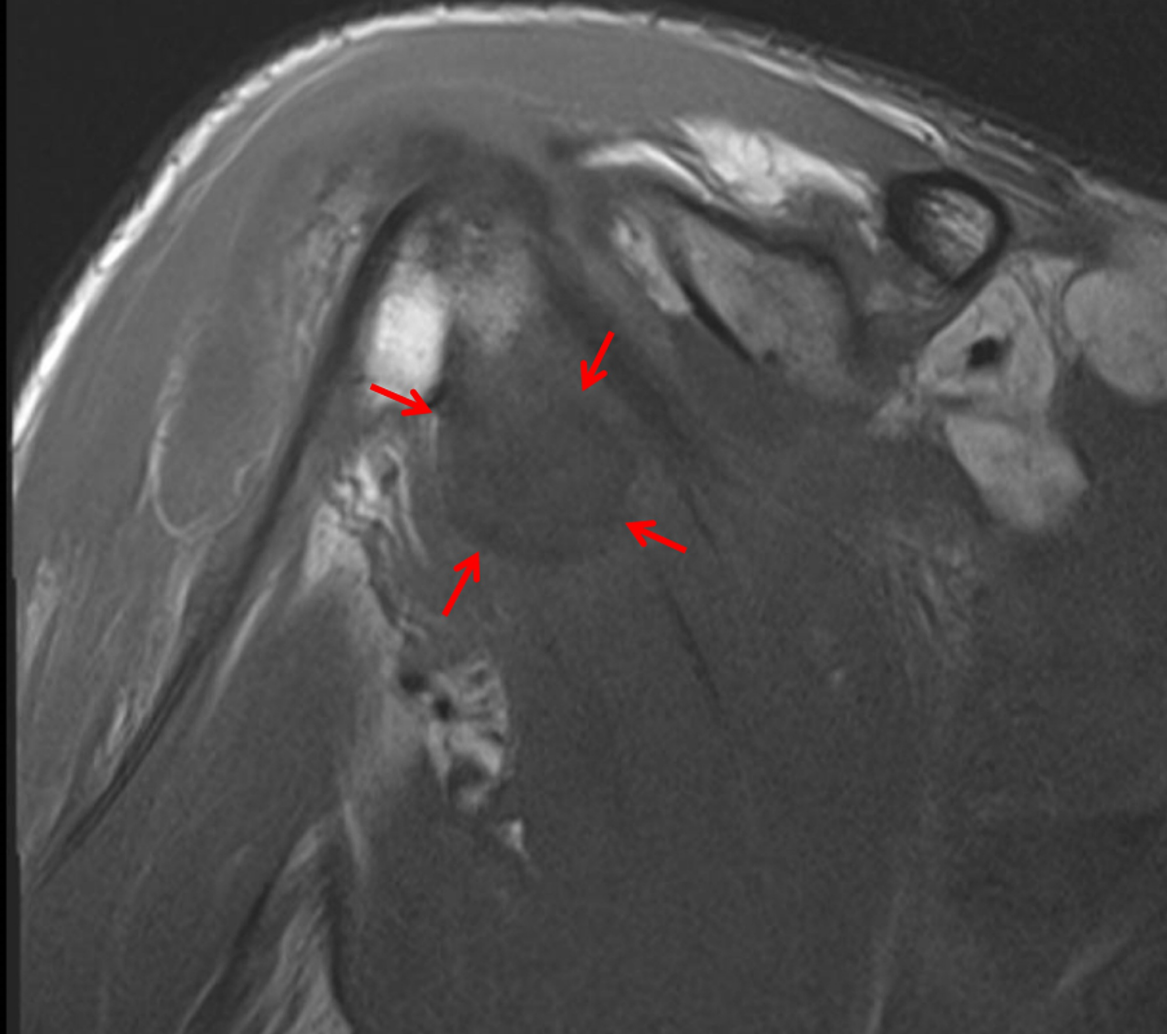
Magnetic resonance imaging (MRI) scan of the right shoulder, coronal section The red arrows indicate the position of the supraspinatus abscess.
